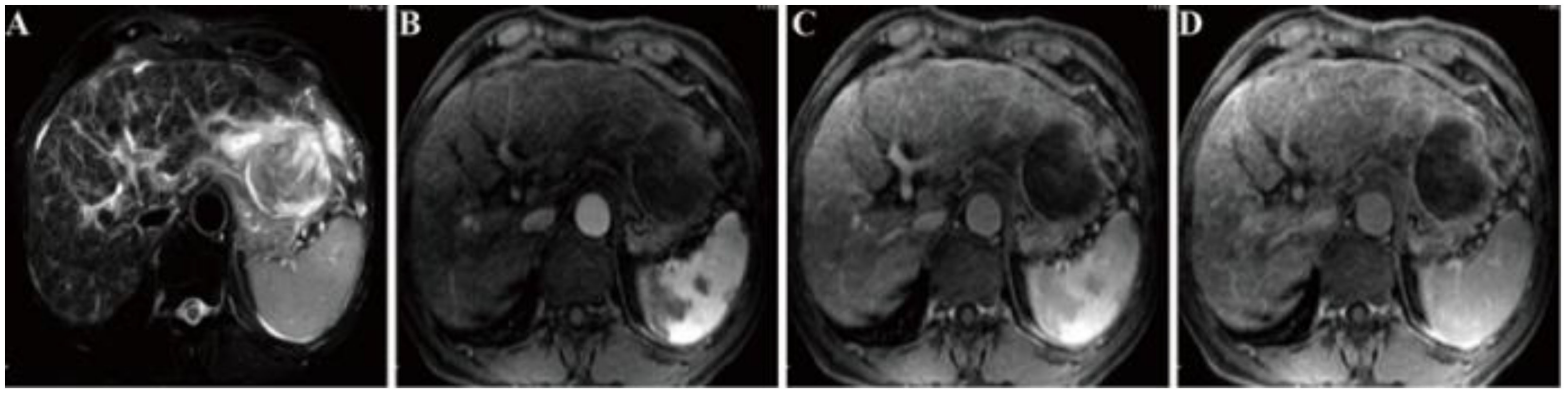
MRI findings (A) An irregular mass with a size of approximately 7.5 cm × 6.9 cm is observed in the left lobe of the liver, exhibiting heterogeneous hyperintensity on T2WI. On contrast-enhanced MRI, the tumor exhibits heterogeneous enhancement during the (B) arterial phase, (C) venous phase, and (D) delayed phase.
